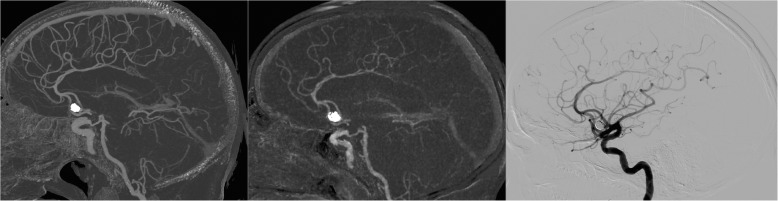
Sixty-seven-year-old male treated with coil occlusion of an anterior communicating artery aneurysm (same patient as in Fig. 1). Follow-up with UHR subtraction CTA (left image), subtraction CTA on a conventional CT system (middle image), and conventional angiography (right image) demonstrated complete occlusion of the aneurysm, with only limited artifacts from the coils
